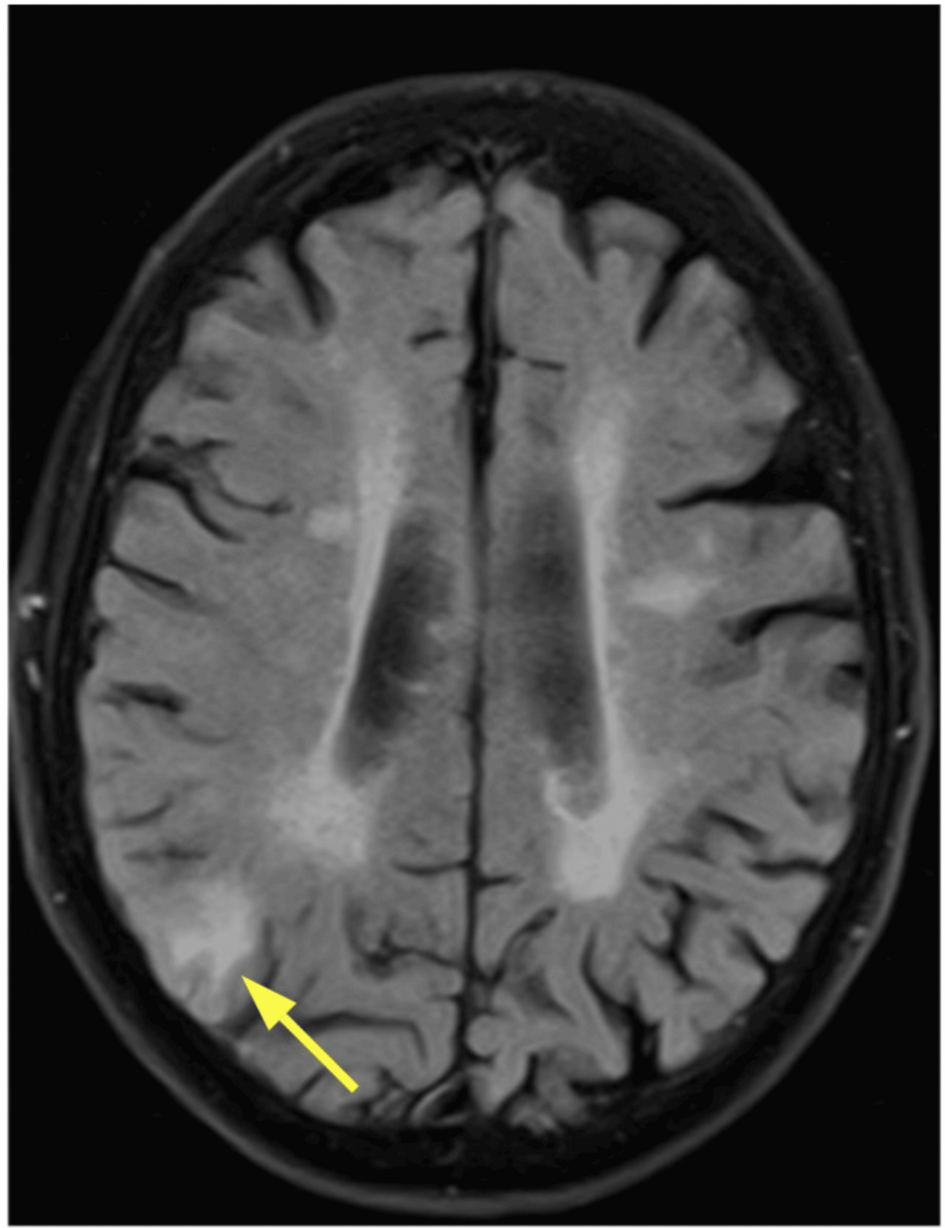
MRI brain: cerebral section Within the right parieto-occipital region is an approximately 2 cm area of cortical/subcortical enhancement. There is surrounding white matter vasogenic edema and local mass effect with effacement of adjacent sulci and right occipital horn. The yellow arrow is pointing to the lesion in the right parieto-occipital region.
